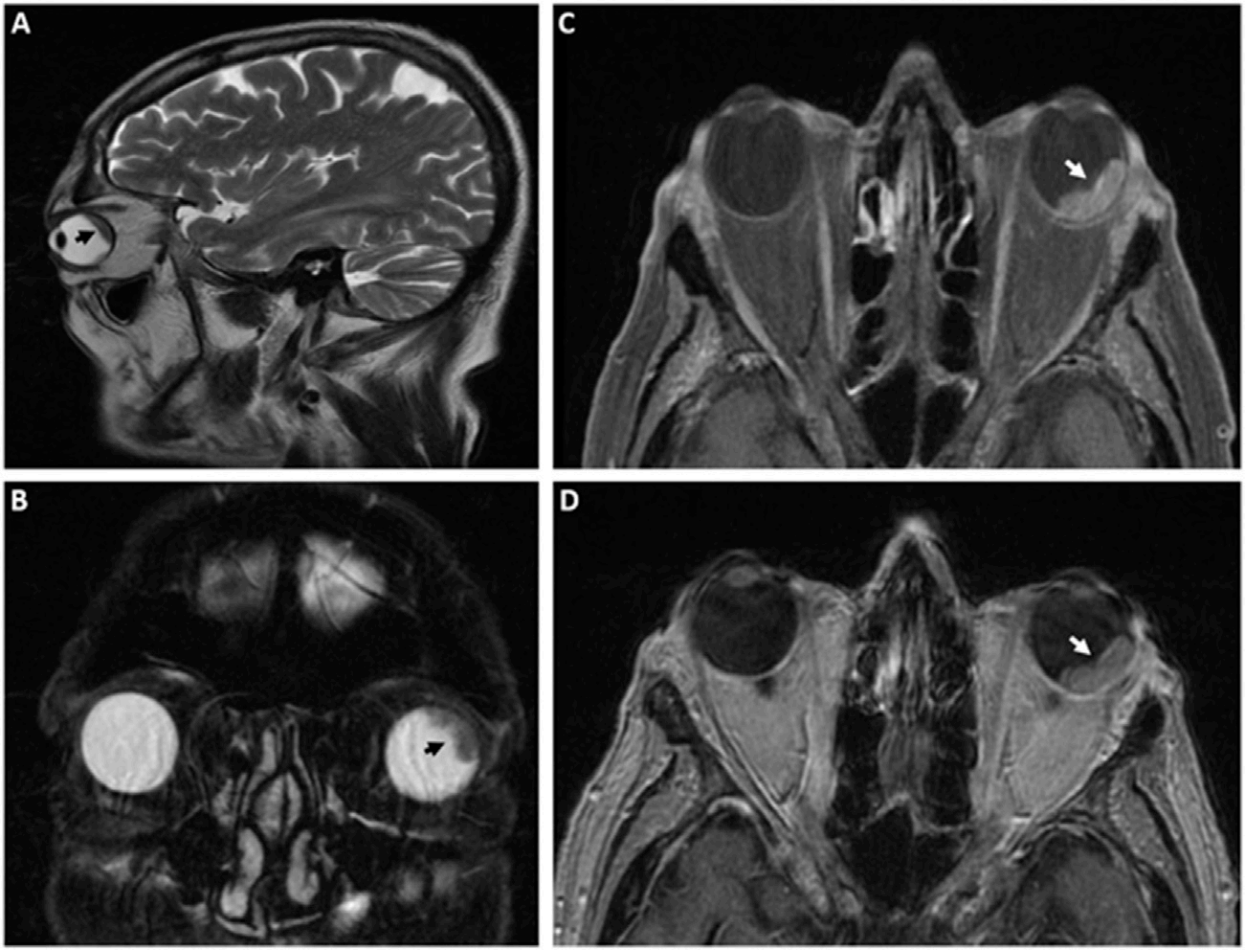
Magnetic resonance imaging (MRI) of the choroidal lesion. A lobulated area of altered signal intensity is observed along the supero-external aspect of the left ocular globe, measuring approximately 19 mm at its largest diameter, with pathological contrast enhancement. MRI sequences include sagittal (A) and coronal (B) T2-weighted images, axial T1-weighted fat-saturated (C), and axial T1-weighted (D) images. The choroidal lesion is indicated by white or black arrows.
